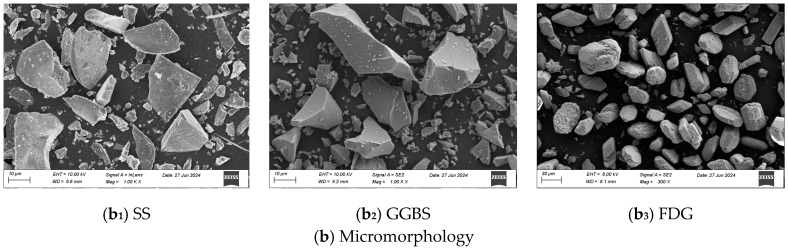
Appearance and micromorphology of SS, GGBS, and FDG.
